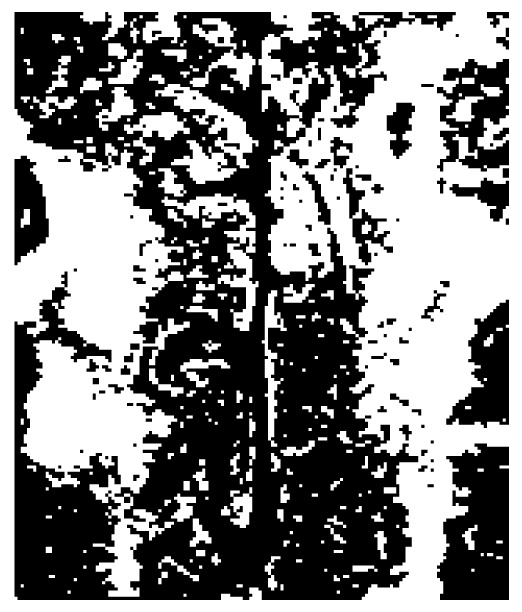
Binary segmentation of the brain MRI using gray-level threshold of 0.3.
